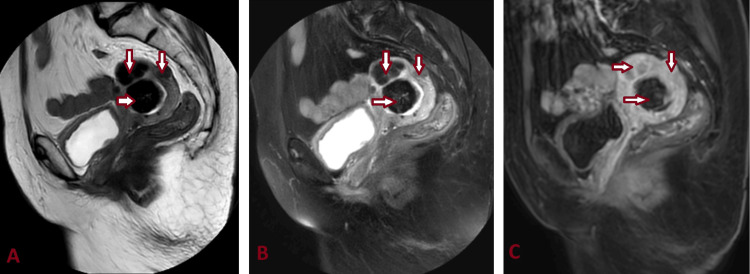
MRI abdomen and pelvis T2 (A), T2-FS (B), and T1-C+ (C) sagittal sections showing no evidence of any focal lesion or significant endometrial collection suggestive of complete resolution. Multiple uterine fibroids (arrows) with calcific degeneration can be seen. FS: Fat saturated; C+: Contrast
